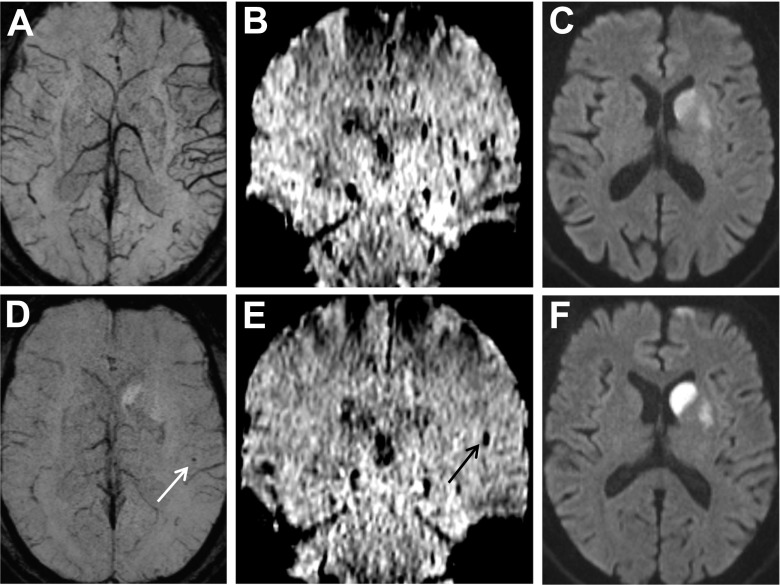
A 72-year-old man with mild aphasia and right-sided hemiparesis (NIHSS score 5). MRI shows left proximal MCA occlusion. On initial SWI, prominent veins in the region of the left Sylvian fissure are seen (a [axial, mIP] and b [coronal]). DWI shows diffusion restriction in the basal ganglia (c). Post-interventional SWI demonstrates a punctate signal drop corresponding to a peri-interventional embolus (d [*white arrow*, mIP] and e [*black arrow*]). The embolus is located outside of the infarcted area as seen on post-interventional DWI (f)
